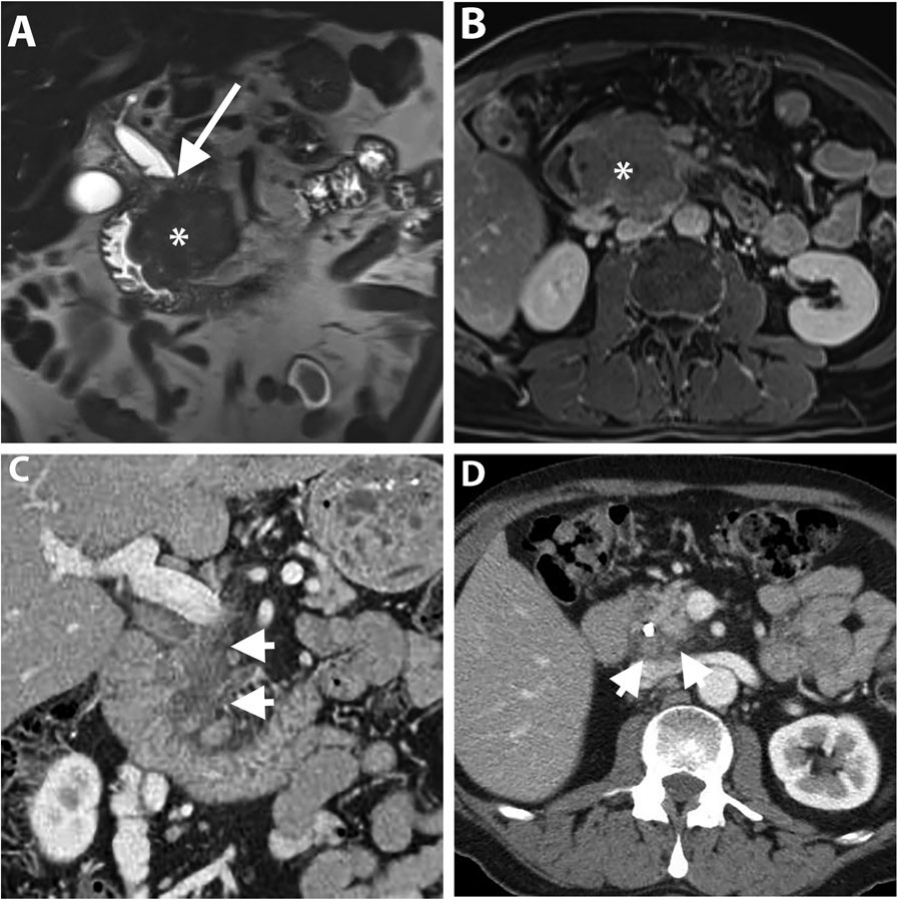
A 64-year-old man with PDAC of the head. Coronal T2-weighted MR image (a) shows a large low-intermdiate signal intensity pancreatic head mass (asterisk) causing upstream CBD dilatation (long arrow). Axial contrast-enhanced T1-weighted image in the portal venous phase (b) shows a hypoenhancing pancreatic head mass abutting SMV. Coronal (c) and axial (d) portal venous phase CT images obtained 2 months later after neoadjuvant therapy show a significant reduction of the tumor bulk (short arrows). The patient subsequently underwent pancreaticoduodenectomy, and pathology revealed extensive neoadjuvant treatment effect on PDAC with only 1 cm residual tumor and negative resection margin. No recurrence has been reported for 3 years
